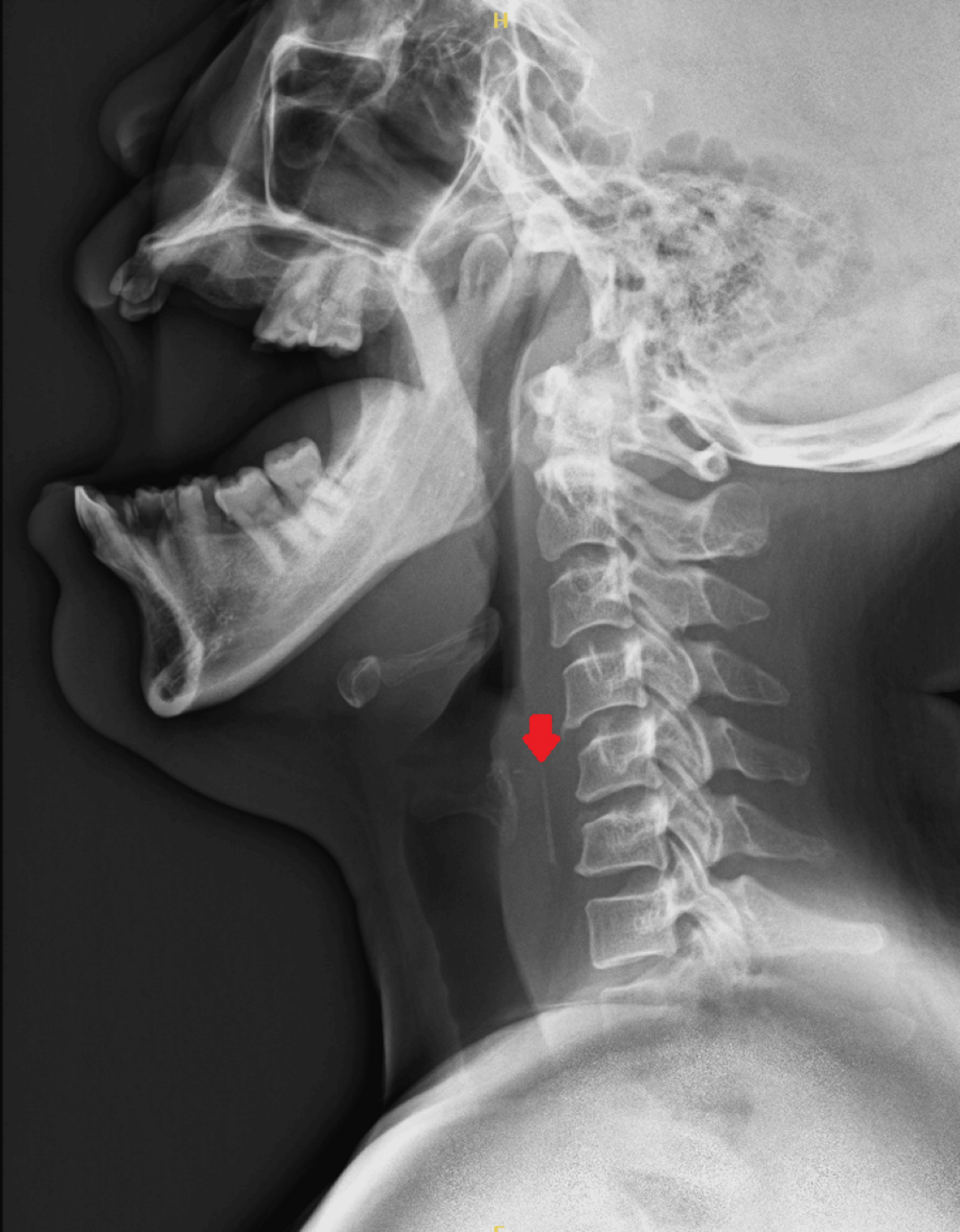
Lateral soft tissue X-ray of the neck revealing suspected foreign body (red arrow).
